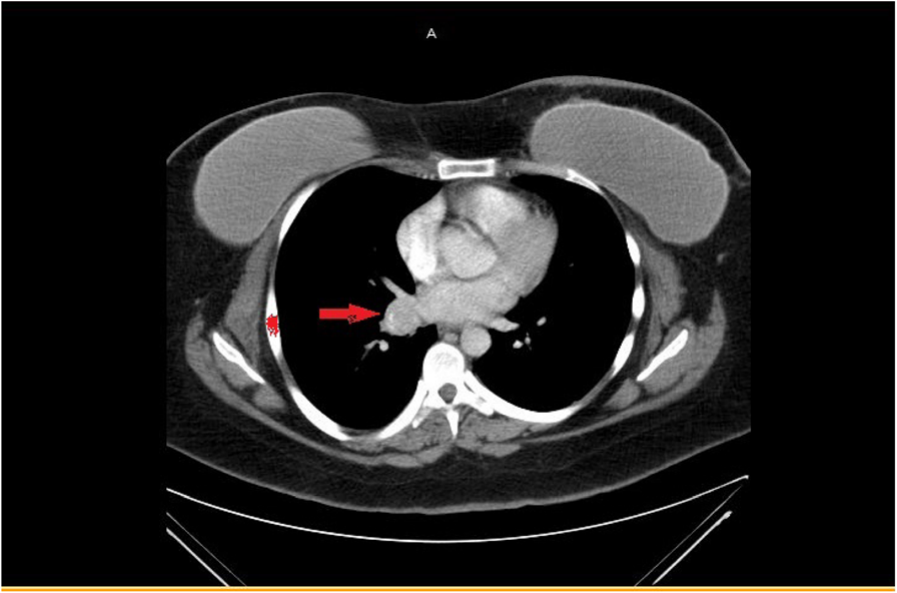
Computed tomographic scan of the chest showing a small lung nodule around the right hilum (*arrow*)
